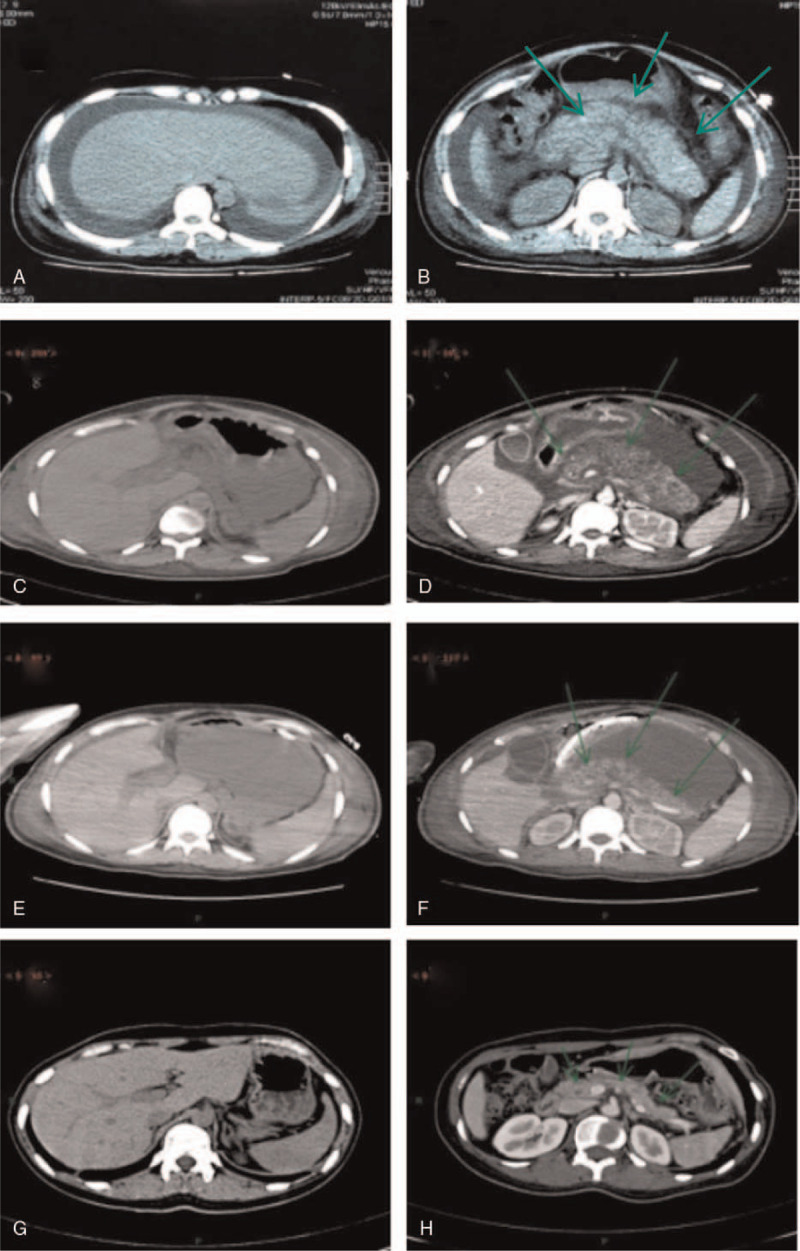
Abdominal computed tomography findings: (A) diffuse decrease of hepatic density. (B) pancreatic swelling, unclear pancreatic duct, blurred peripheral fat spaces, and peripheral foci of exudation. (C) normal size, shape and density of the liver. (D) obvious pancreatic swelling with reduced pancreatic parenchymal density and lytic necrosis. (E) homogeneous density of liver parenchyma. (F) reduced volume and uniform density of pancreas. (G) normal size, shape and density of the liver. (H) uniform pancreatic density.
